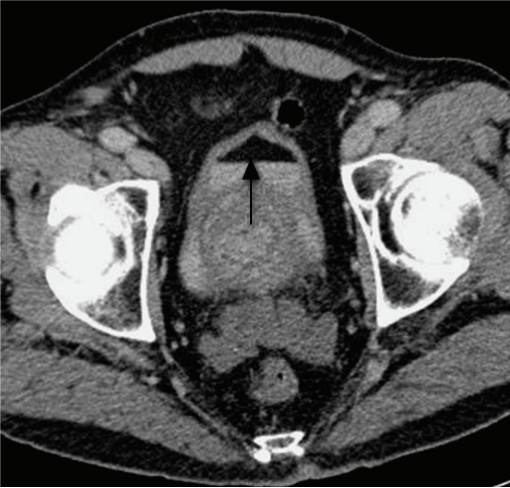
Axial section of contrast-enhanced CT of the bladder shows a fat-fluid level (black arrow) within the bladder, consistent with chyluria.
